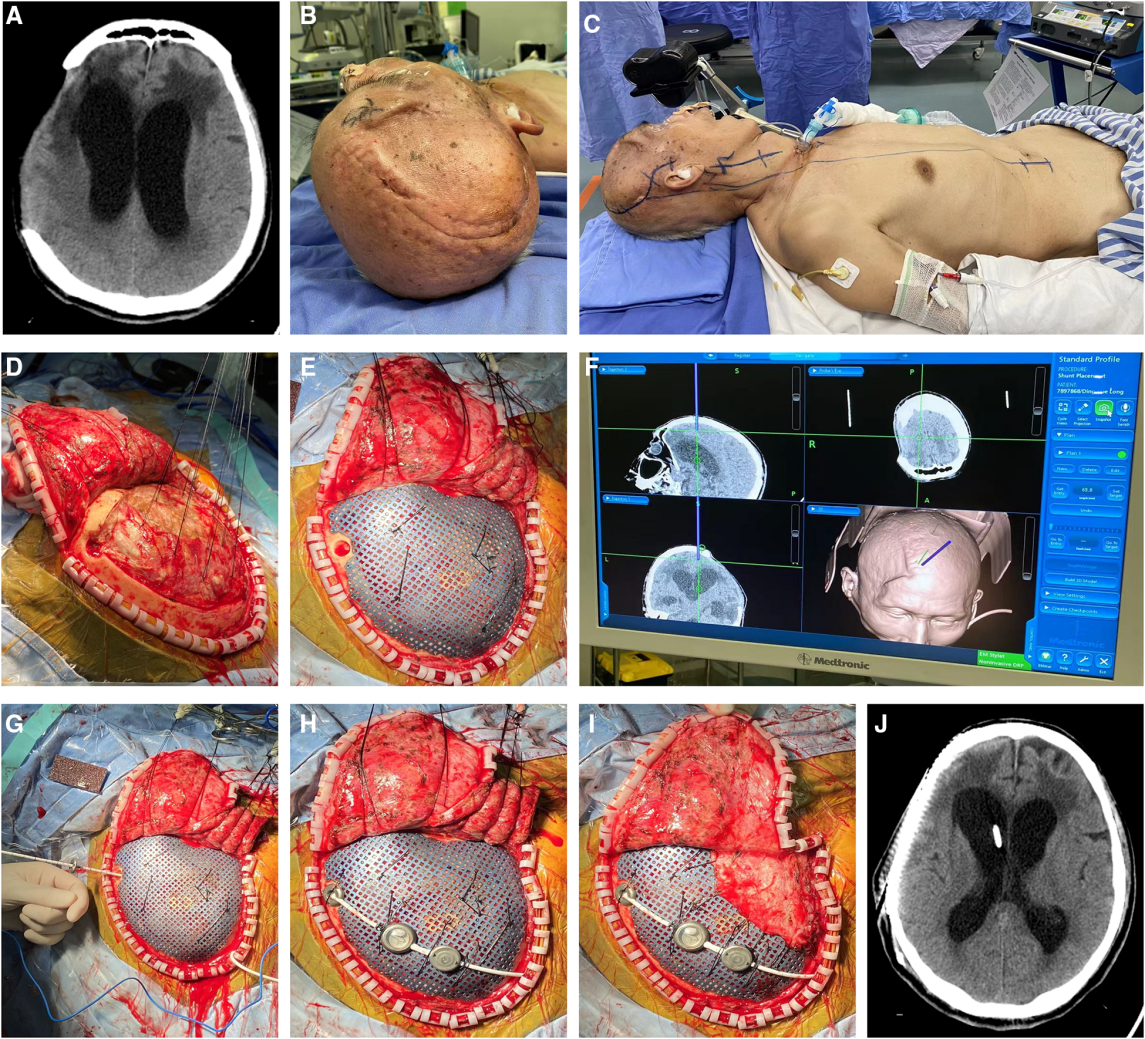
(A) Head CT scan showed right cranial defect and dilation of the ventricle system. (B,C) According to strategy 1, cranioplasty was performed first. Shunt valve pressure was set at 160 mm H_2_O before the surgery. (D) Potential epidural space disappeared after suspending with silk thread between titanium mesh and dura mater. (E) The entry point was located by the neuronavigation system on the right frontal bone. (F–I) Then, ventricular puncture was implemented and the shunting tube was connected. (J) No subdural/epidural fluid collection or hemorrhage was revealed by postoperative head CT.
